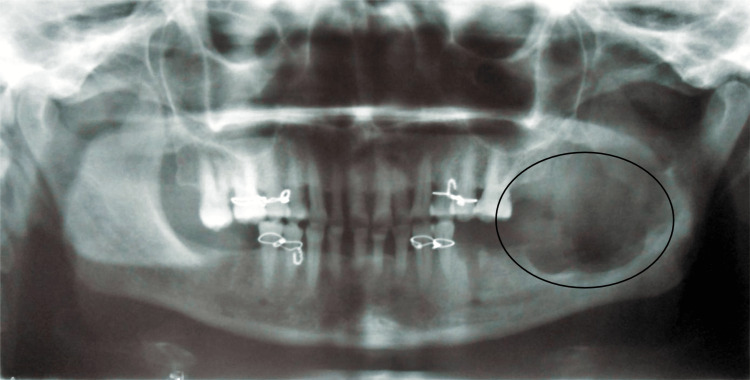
Post-operative radiograph showing surgical defect involving the left side of the mandible extending from 35 to the ramus region
